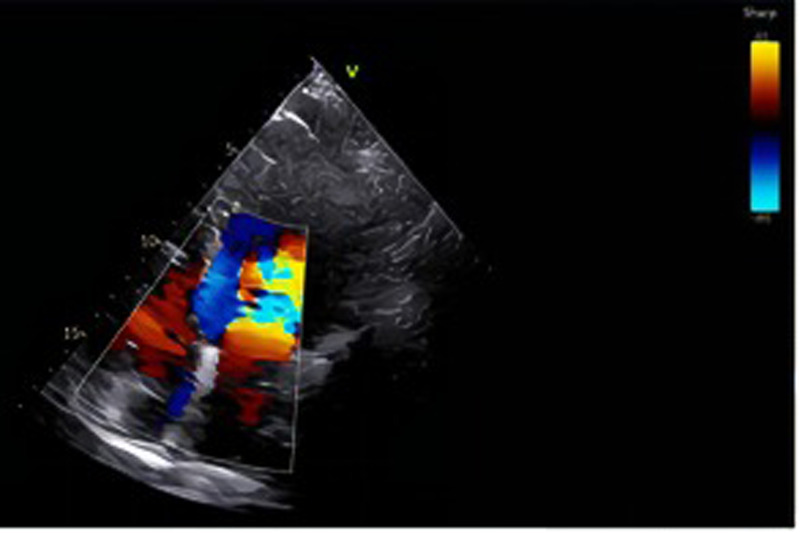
In the lower left to mid region of the image, a distinct mosaic flow signal comprising red, blue, and yellow is observed, located near the posterior wall of the atrium or the pulmonary vein inflow area. Under physiological conditions, color Doppler signals are typically confined to the valvular orifice and adjacent flow regions. However, in this case, the jet deviates from the valvular axis and traverses obliquely upward, suggesting the presence of an abnormal flow pathway from a non-physiological direction. This imaging feature raises suspicion of a pulmonary arteriovenous malformation (PAVM), which may facilitate a right-to-left shunt.
